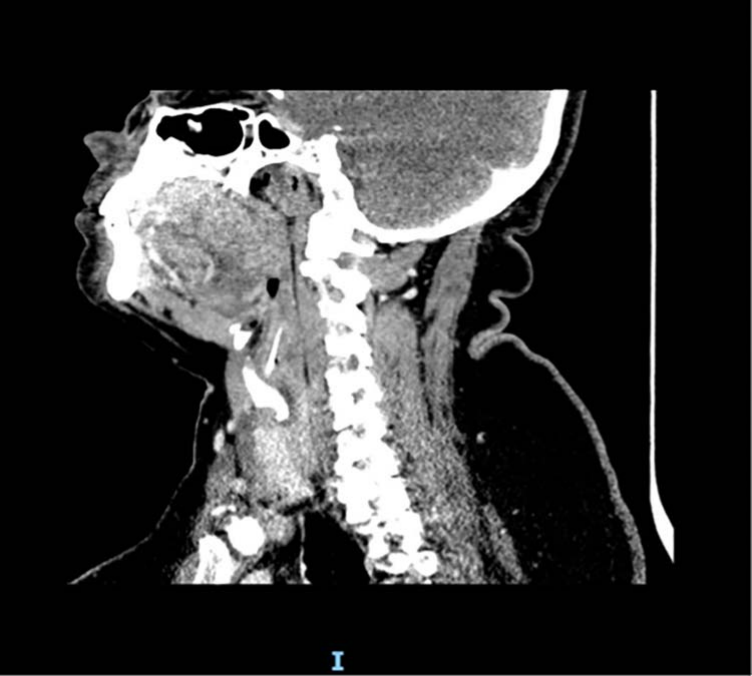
CT scan showing location of fish bone.
